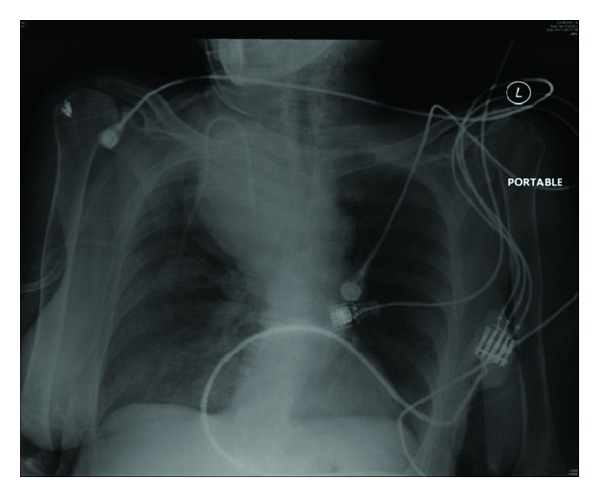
Postprocedural chest X-ray showing right mediastinal hematoma. Thoracic and vascular surgery team was consulted immediately and the decision was made to emergently take the patient to the operating room for evacuation of a mediastinal hematoma. Central and arterial access was obtained and transfusion of packed red blood cells (PRBC) was started. The hemodynamic status improved.
